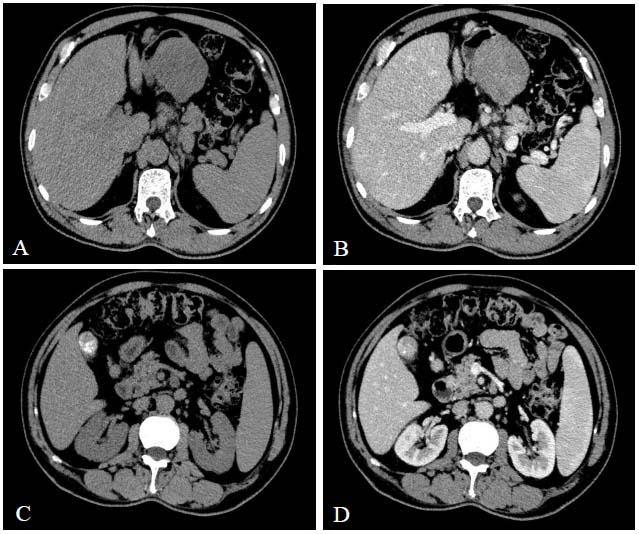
CT cross section of enlarged lymph nodes. The red arrows in (A) and (B) show enlarged lymph nodes near the gastric angle, and the enhanced scan shows obvious enhancement. The blue arrows and purple arrows in (C) and (D) show enlarged lymph nodes near the hepatogastric space and retroperitoneal large blood vessels, respectively, and the enhanced scan shows obvious enhancement.
